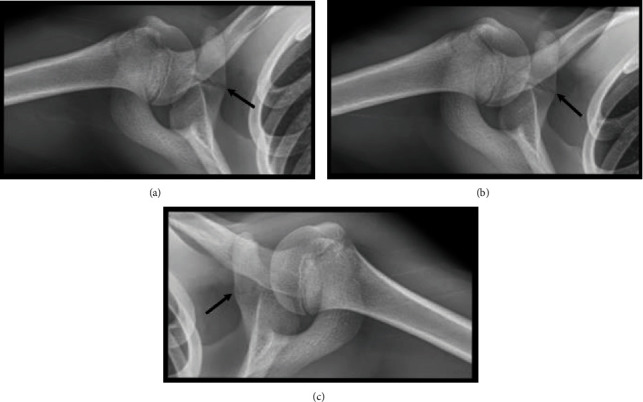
(a) Radiograph of injured shoulder taken at first clinical visit (12/27/2018) (7.5 weeks after the injury). (b) Radiograph of injured shoulder taken at the second clinical visit (1/31/2019) (approximately 11 weeks after the index injury). Note that the lucency extends across the entire coracoid. (c) Radiograph of contralateral (noninjured) shoulder taken at second clinical visit. Note that the lucency at the medial aspect of the coracoid is much less obvious when compared to the injured side.
